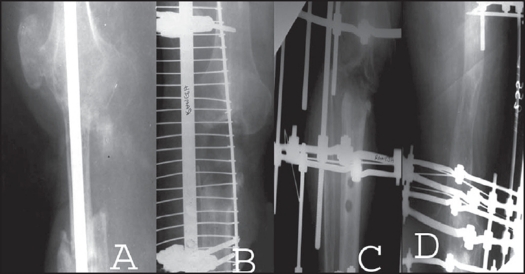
A) Postoperative x-ray of the patient showing intercalary allograft threaded over an intramedullary nail. B) Sequestered graft removed and tubular external fixator applied. C, D) Telescoping of the bone ends with simultaneous lengthening of femur
